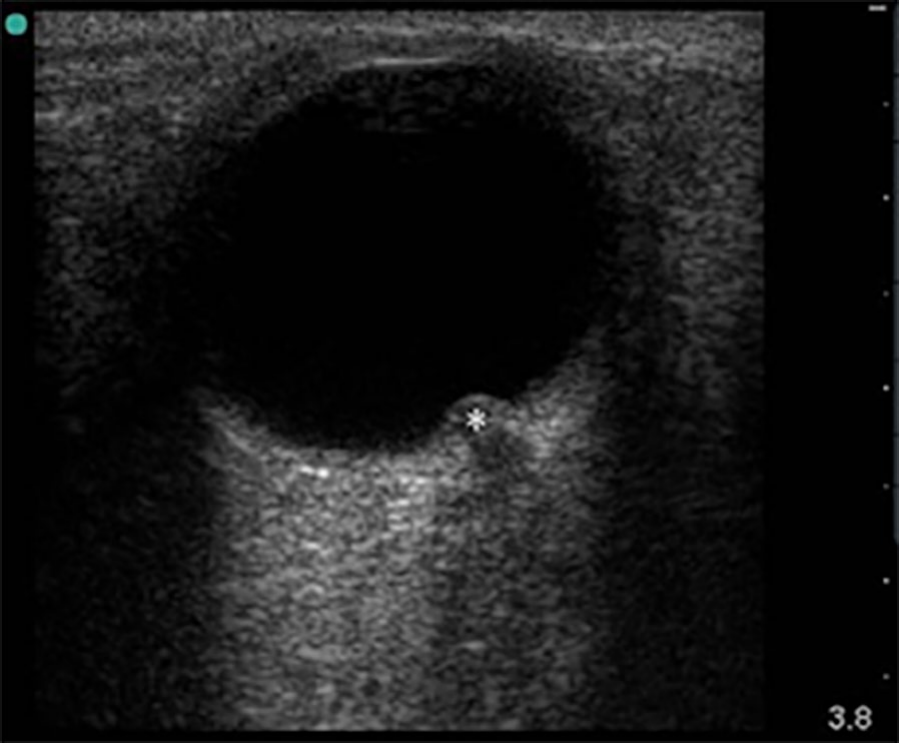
Elevation of the optic disc, marked with a star on B scan ocular ultrasound
